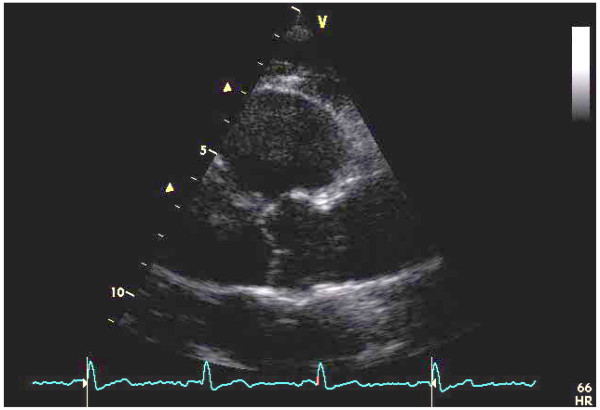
Aortic root and proximal ascending aorta in parasternal long-axis view by 2-D echocardiography. By parasternal approach, a long-axis view was modified in order to maximize the imaging of the aortic valve, the sinuses of Valsalva, the sino-tubular junction and the proximal ascending aorta at end-diastole. The probe was thus swept in order to make the whole aortic root as perpendicular as possible to the ultrasound beam. Gain settings, compensation and dynamic ranges were adjusted to optimize aortic wall definition.
